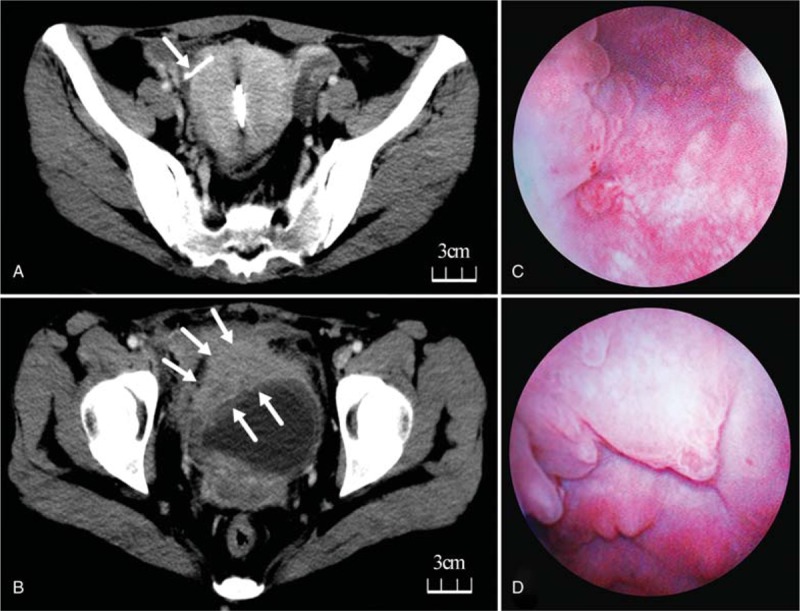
(a, contrast-enhanced CT): (1) Anterior bladder wall was extensively thickened (2.3 cm) and adjacent tissues were involved, (2) border of the posterior bladder wall could not be clearly distinguished from the uterus, (3) multiple lymph nodes were enlarged around the abdominal aorta, bilateral internal, and external iliac vessels (IUD was not mentioned by this CT report). (b, contrast-enhanced CT): A portion of one of the 2 implanted IUDs had migrated beyond the right uterus wall and was closely adjacent to the bladder. (c, d): Enormous intravesical benign hyperplasia were showed by cystoscopy.
